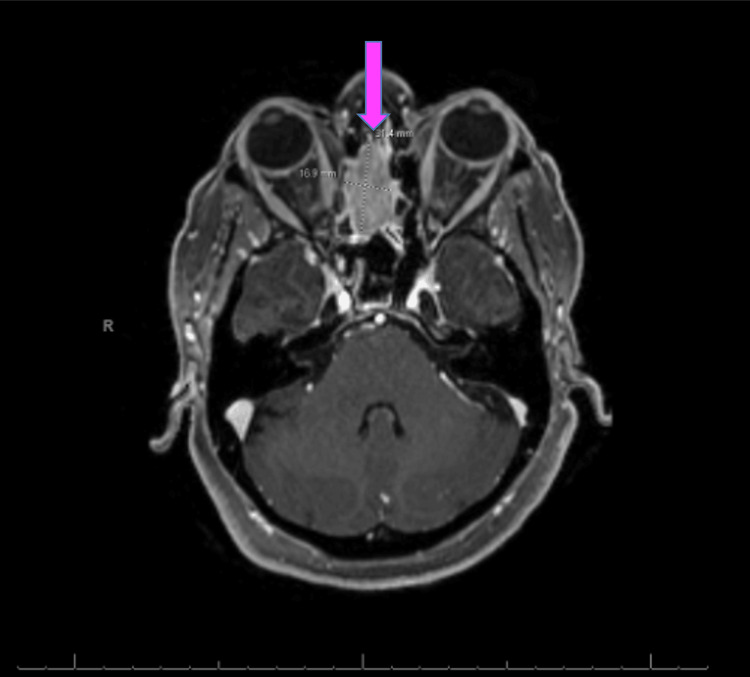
MRI of the brain confirmed the upper nasal cavity mass
